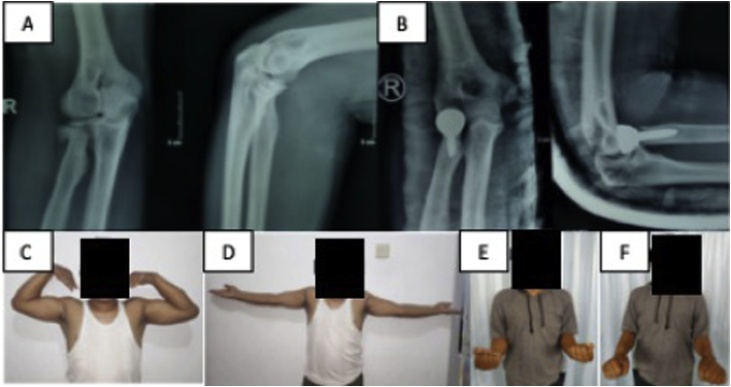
A Pre-operation AP/Lateral X-Ray of the right elbow from the first patient showing displacement and comminution of the radial head. Fig. 1B Post-operation AP/Lateral X-Ray of the right elbow from the same patient. The injury was treated with radial head arthroplasty. Fig. 1C–F Active ROM evaluation of the same patient; elbow flexion of 110°; elbow extension of 10°; elbow supination of 85°; elbow pronation of 75°.
